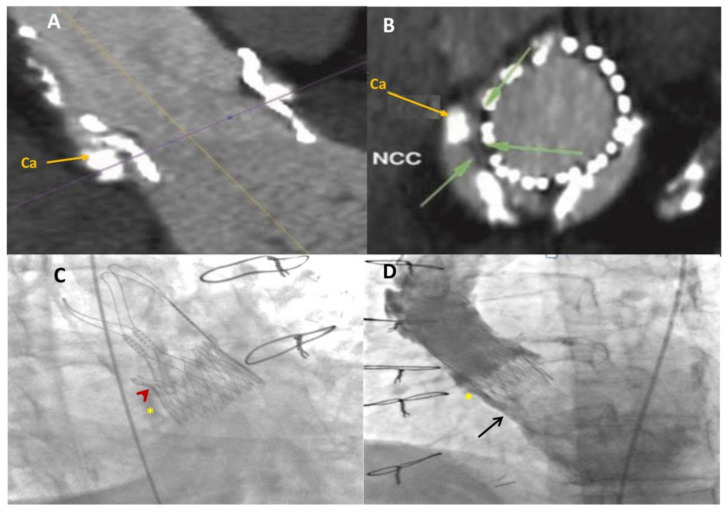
Multidetector computed tomography angiography (MDCTA) scans with acquired frames in the axial (A) and coronal (B) views showing the location of the leakage (green arrows) and the calcified nodule (Ca, yellow arrow) located at the level of the regurgitant jet at the non-coronary aortic cusp; (C) fluoroscopic image showing the self-expandable aortic bioprosthetic valve in place and the calcified nodule (yellow star) and its indentation (red arrowhead) on the non-coronary cusp; (D) baseline ascending-aorta angiogram (LAO projection) confirming severe paravalvular regurgitation (black arrow) of the self-expandable bioprosthetic aortic valve in close proximity to the calcified nodule (yellow star) at the non-coronary cusp. NCC, non-coronary cusp; AA, ascending aorta.
